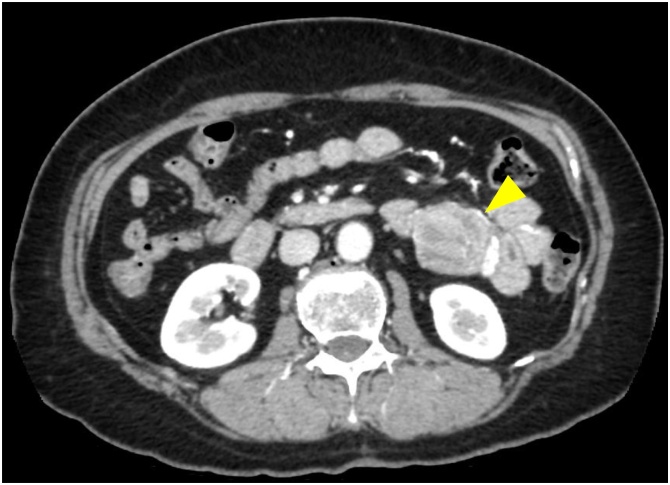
A contrast-enhanced CT scan shows a 40 mm well-enhanced, round tumor that was located at the jejunum (arrow head).
